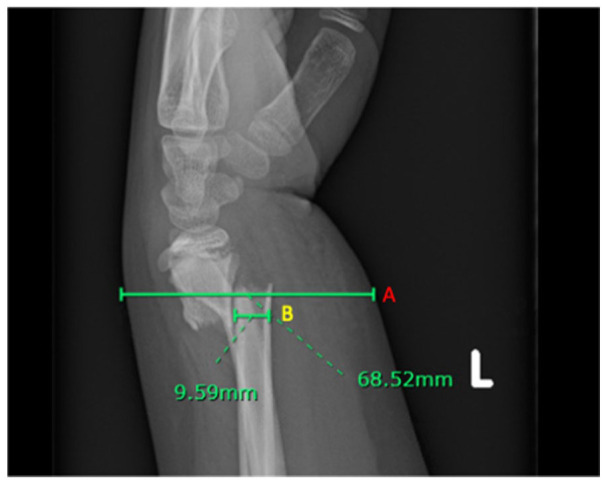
Sagittal radiograph of a displaced distal third both bone fracture in a skeletally immature patient. (A) indicates the soft tissue width at the fracture site, and (B) indicates the radius width at the fracture site.
